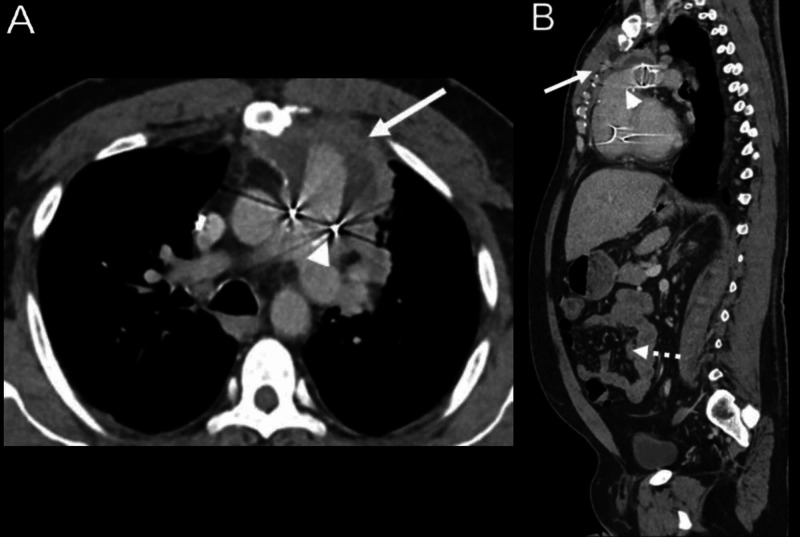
Contrast-enhanced CT of the chest, abdomen, and pelvis showing mediastinal abscess. Axial and sagittal postcontrast CT through the chest reveals pulmonary outflow tract reconstruction evident postsurgical material and resultant streak artifact (arrowhead). Anterior to the pulmonary artery outflow tract, hypodense rim enhancing fluid collection is seen suggestive of abscess (A and B, arrows). Mild thickening of mid small bowel loops with surrounding fat stranding is suggestive of enteritis (B, dashed arrow). Urinary bladder wall thickening and inflammatory changes represent cystitis.
